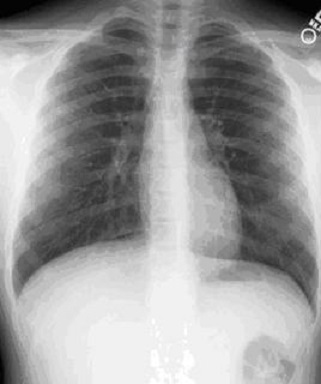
Radiographie thoracique normale
